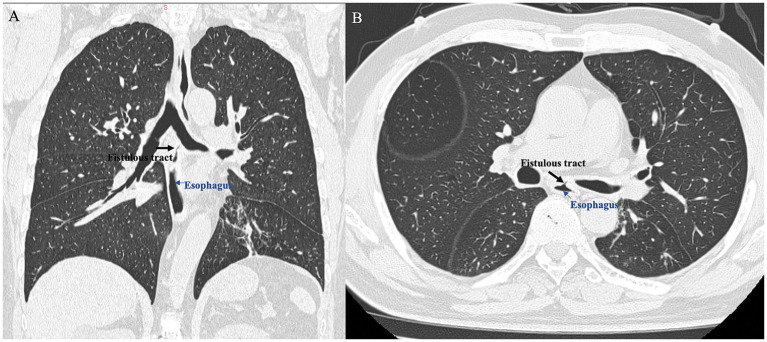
Preoperative thoracic imaging. (A) Coronal and (B) axial chest computed tomography demonstrating a direct, upward fistulous tract (black arrow) between the mid-esophagus (blue arrow) and the left main bronchus, consistent with the Braimbridge and Keith Type II congenital bronchoesophageal fistula.
